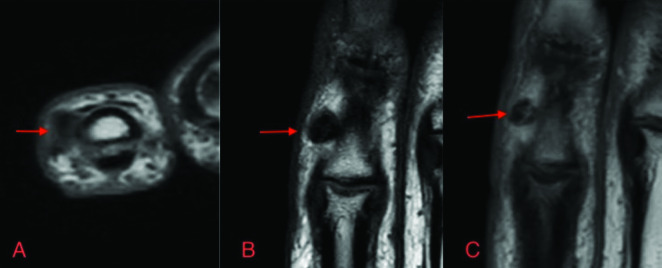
MRI of the lesion. Central hyperintensity on axial T2 (A). Peripheral and central hypointensity on T1 (B). Discrete central contrast enhancement on T1 (C).
